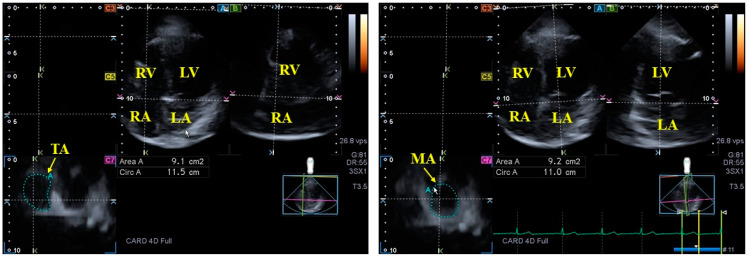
Three-dimensional (3D) speckle-tracking echocardiography-derived two-dimensionally projected views of the tricuspid (TA) (left panel) and mitral annuli (MA) (right panel). After 3D echocardiographic dataset acquisitions, following adjustments on apical four-chamber (A) and two-chamber (B) long-axis views, TA/MA-optimized images are presented in C7 cross-sectional views (C7). The yellow arrows represent the TA/MA plane. Abbreviations: LA = left atrium, LV = left ventricle, RA = right atrium, RV = right ventricle, Area = TA/MA area, Circ = TA/MA perimeter.
